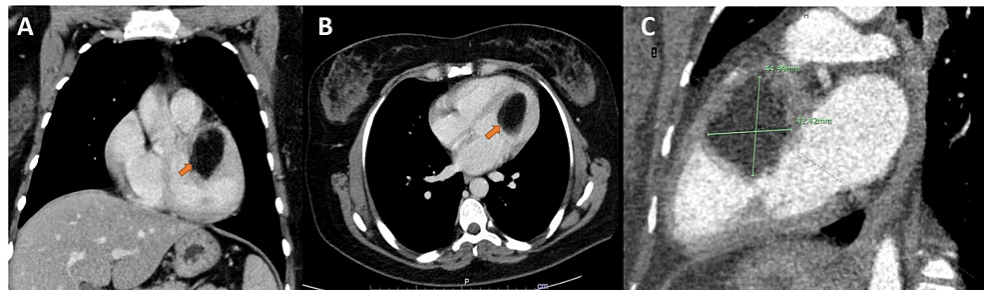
Computed tomography scan Lipoma (orange arrows) on coronal (A), axial (B), and posterior oblique (C) views.
